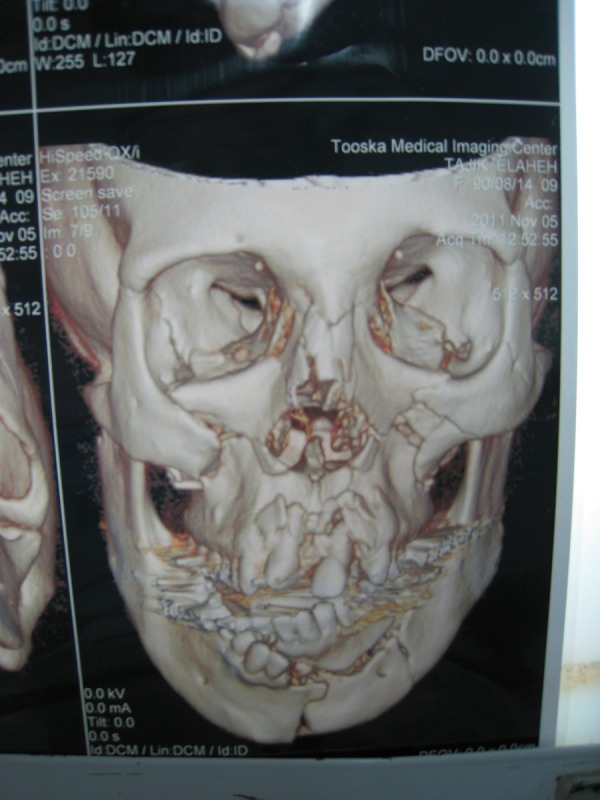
3D reconstruction CT of a Patient With Panfacial Fractures. Note Nasoethmoidorbital Fracture, LeFort 1 and 2, Zygoma, Maxilla, Alveolar, Mandibular and Dental Fractures
